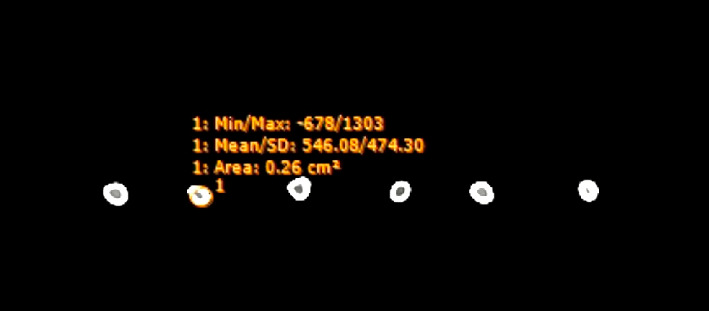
2D image with mean/SD of CT value.
